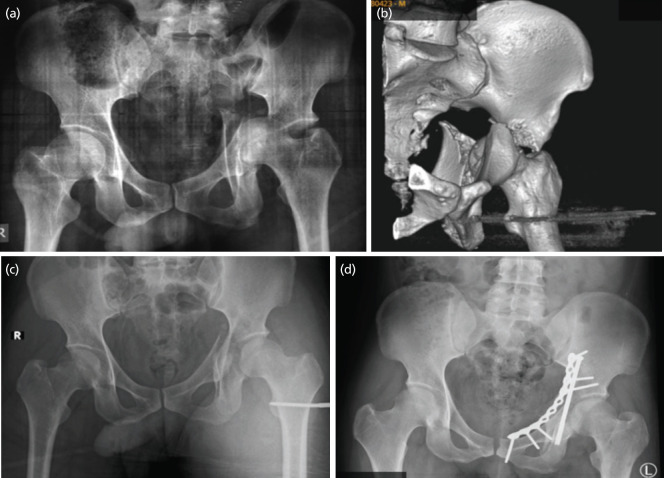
(a) Initial post-trauma pelvis AP radiograph showing fracture displacement and hip dislocation. (b) Pre-operative pelvis AP radiograph showing final reduction on vector traction 15kg. (c) Post-operative pelvis AP radiograph.
